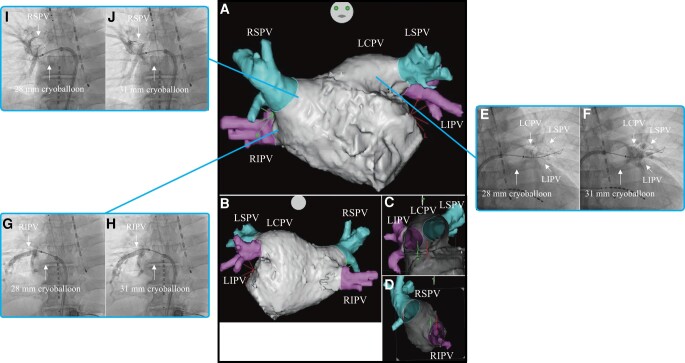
Three-dimensional images obtained through a multi-slice CT scan of Case 1 in the anteroposterior (*A*), posteroanterior (*B*), right-lateral (*C*), and left-lateral (*D*) views. Venogram of the LCPV during cryoballoon ablation using the 28 mm (*E*) and 31 mm (*F*) modes. Venogram of the RIPV during cryoballoon ablation using the 28 mm (*G*) and 31 mm (*H*) modes. Venogram of the RSPV during cryoballoon ablation using the 28 mm (*I*) and 31 mm (*J*) modes. CT, computed tomography; LCPV, left common pulmonary vein; RIPV, right inferior pulmonary vein; RSPV, right superior pulmonary vein.
